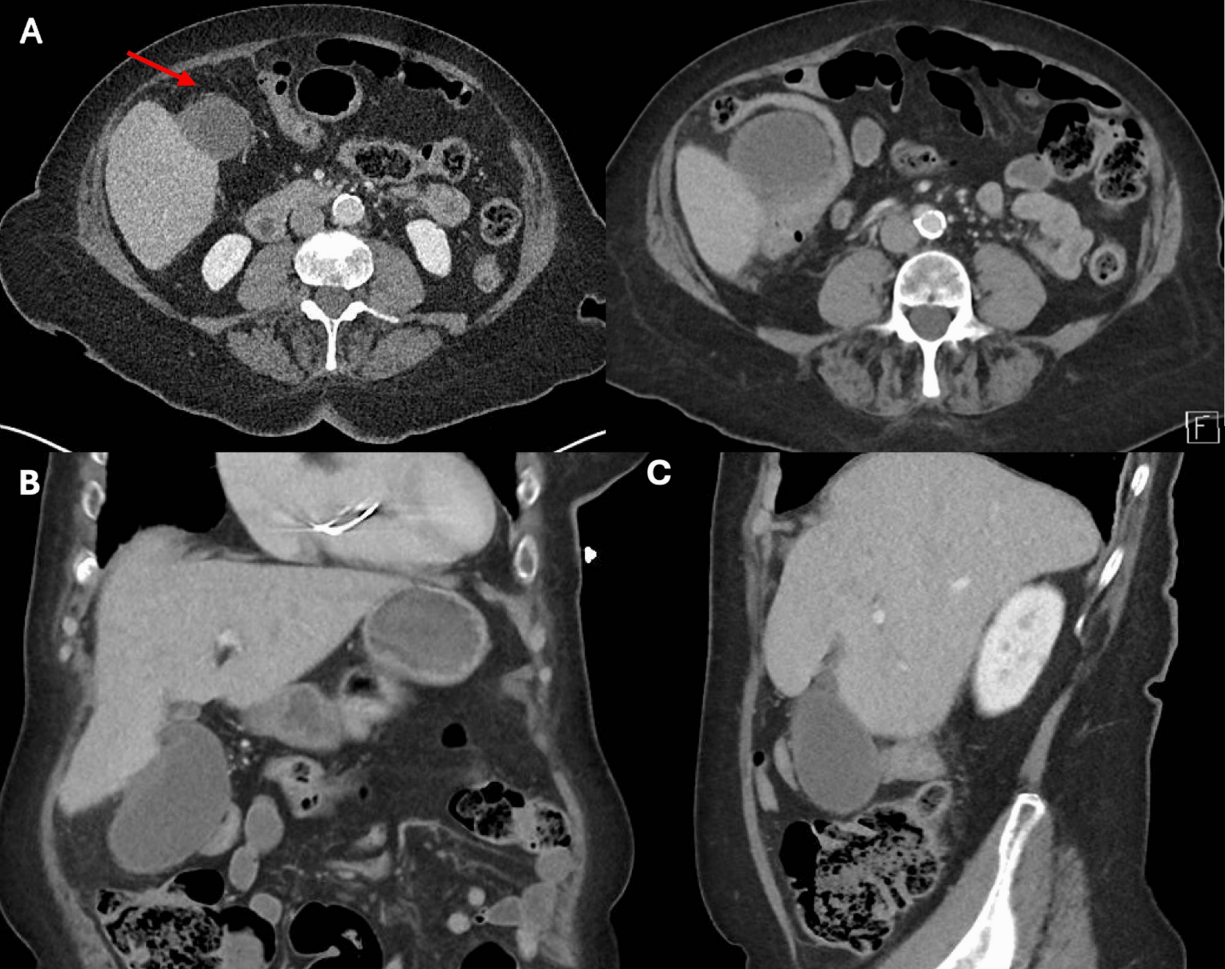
Contrast-enhanced CT of the abdomen and pelvis showing a distended gallbladder. A thickened gallbladder wall measuring 4 mm was noted. Mild fat stranding can be seen along the body of the gallbladder. No radiopaque cholelithiasis was noted. A. Axial images of the gallbladder showing mild fat stranding (arrow). B. Coronal image of the gallbladder showing a distended gallbladder and gallbladder wall thickening. C. Sagittal image of the gallbladder showing a distended gallbladder.
